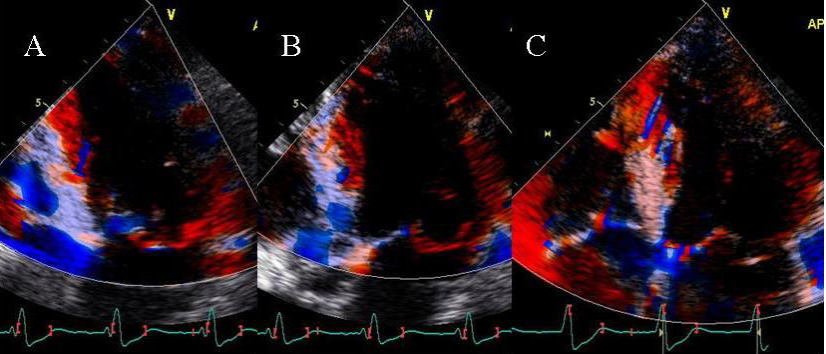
Strain images obtained in apical 4 views with an AV delay of 220 ms and LV pre-excitation of 20 ms (A), AV delay of 220 ms and VV delay of 0 ms (B) and AV delay of 220 ms and RV pre-excitation of 20 ms (C). Note improved strain in the interventricular septum, LV lateral wall and right ventricular free wall during RV pre-excitation.
